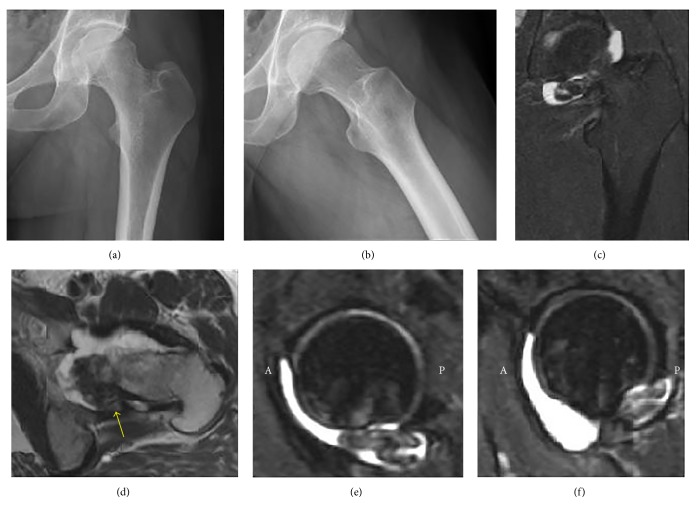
Preoperative anteroposterior (a) and frog-leg radiographs (b) of the left hip in Case 2 demonstrate a cam deformity. Preoperative coronal T2-weighted fat-saturated (c), axial T2-weighted (d), and sagittal T2-weighted fat-saturated (e, f) magnetic resonance sequences with intravenous contrast reveal a multilobulated low signal lesion (arrow in d) measuring approximately 4.0 × 1.1 × 3.4 cm within the posterior/inferior aspect of the hip joint, with an associated large joint effusion. A = anterior; P = posterior.
